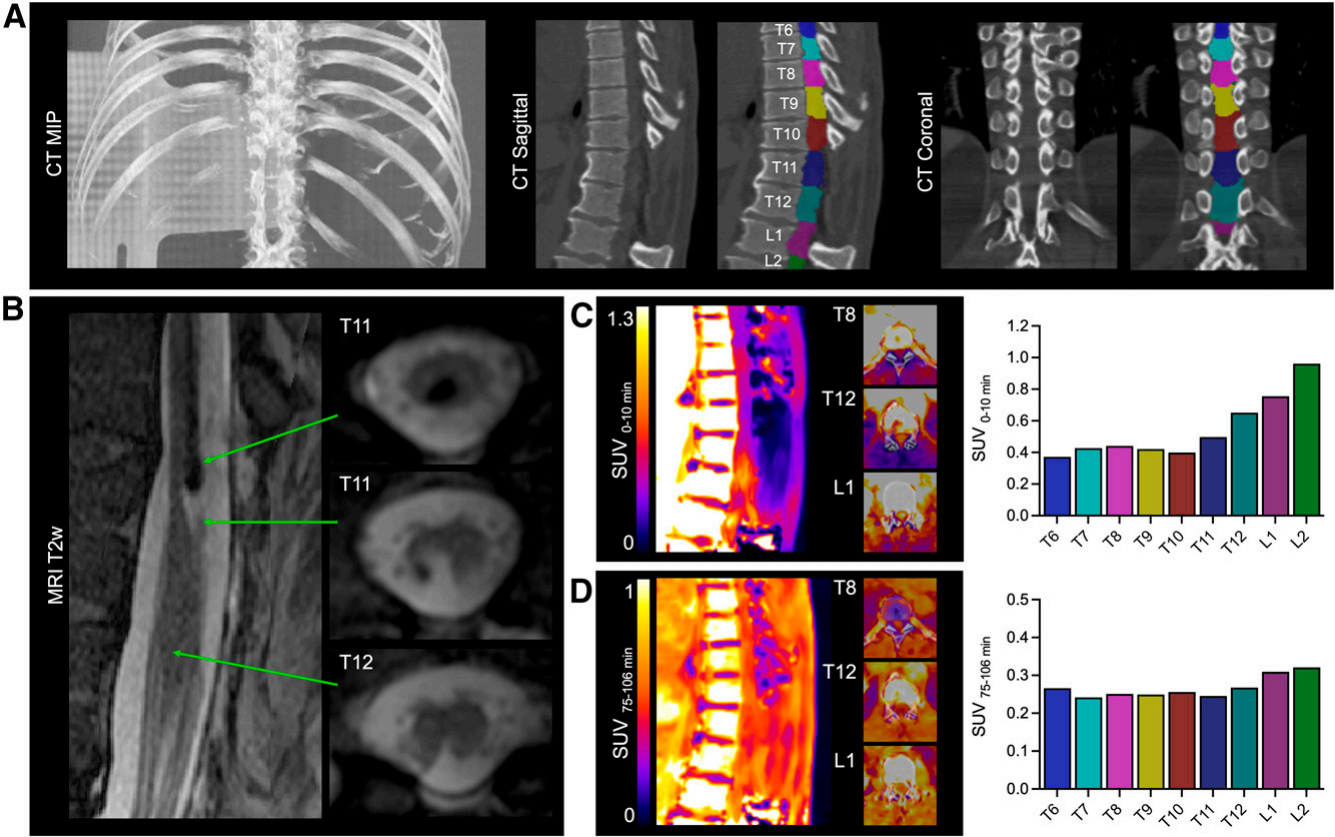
[^18^F]3F4AP in a human subject after mild incomplete SCI. (A) Maximum-intensity projection (MIP), sagittal, and coronal CT images showing laminectomies at T10–T12. Selected regions of interest for segmentation of spinal cord are shown for both sagittal and coronal views. (B) T2-weighted (T2w) MR sagittal and axial images showing spinal lesion. (C) Early PET sagittal images showing high PET signal in cord around injury and quantification 0–10-min SUV at selected spinal segments. (D) Late PET sagittal images showing increased PET signal in cord around injury and quantification of 75–106-min SUV at selected spinal segments. For both early and late PET, axial images at T8, T12, and L1 are shown.
